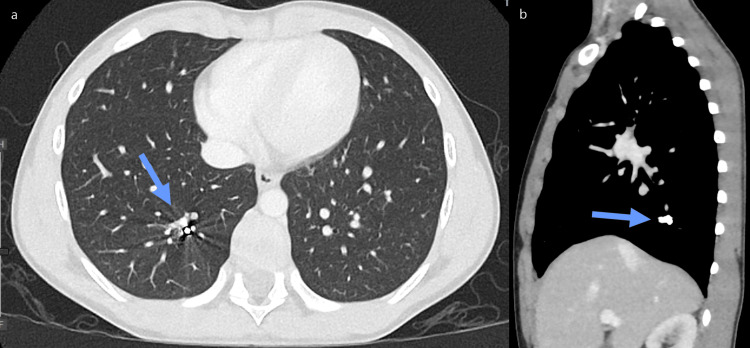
(a) Axial CT of the chest: Two small circular radio-opacities (arrows) located in the posterior basal segment of the right lower lobe. (b) Sagittal CT chest. Image credit: Dr. Lena Naffaa CT: Computed tomography
